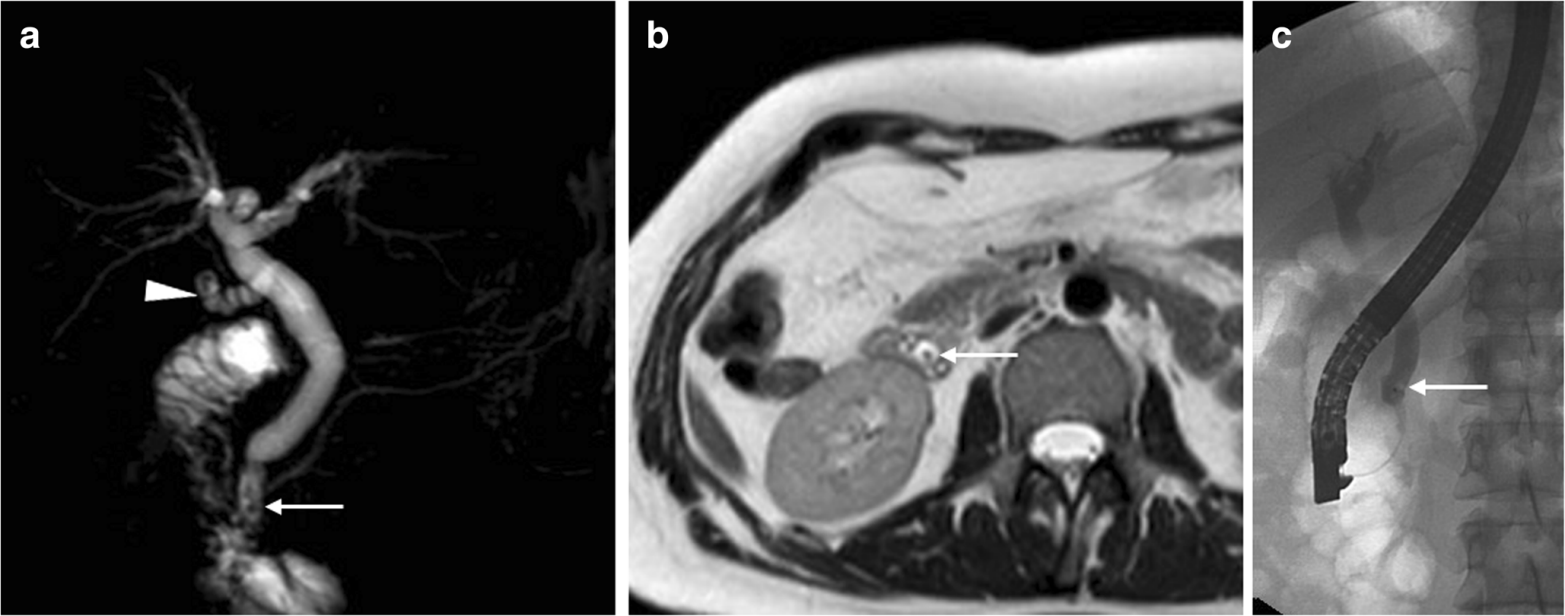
Residual lithiasis in the common bile duct (CBD) following recent (10 days) laparoscopic cholecystectomy performed at another hospital. MRCP (a) and axial T2-weighted (b) MR images showed unremarkable cystic duct remnant (*arrowhead*) and mildly dilated CBD with small dependent filling defects (*thin arrows*). Residual lithiasis was confirmed at ERCP (*thin arrow* in c) and treated by sphincterotomy and extraction using a Dormia basket
